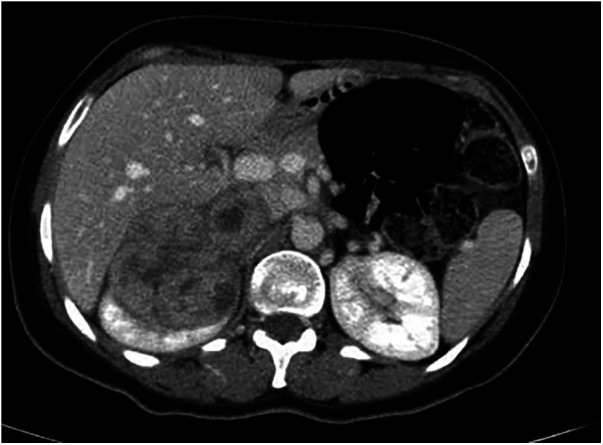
Ct, CT findings for case 1.
